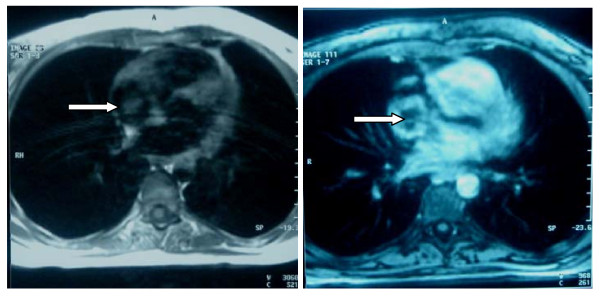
MRI showing a mass occupying the right atrium.
